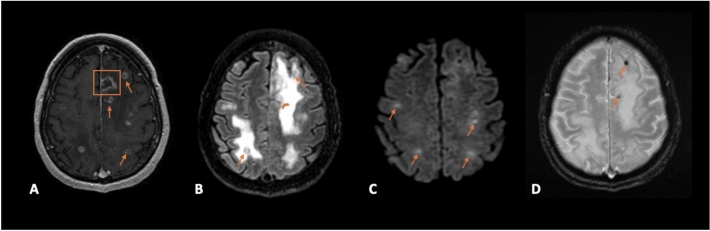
Example of a patient with EBV-associated brain lymphoma. A Multiple lesions demonstrating ring enhancement (arrow) and necrotic, heterogeneous enhancement (square), all with a cortico-subcortical distribution; B On FLAIR imaging, the lesions appear hypointense (arrow) or isointense (arrowhead), accompanied by significant perilesional edema; C Diffusion-weighted imaging (DWI) with a b-value of 1000 (b1000) shows restricted diffusion within the lesions (arrow); D T2-weighted gradient echo imaging reveals hemorrhagic components in some of the lesions (arrow)
